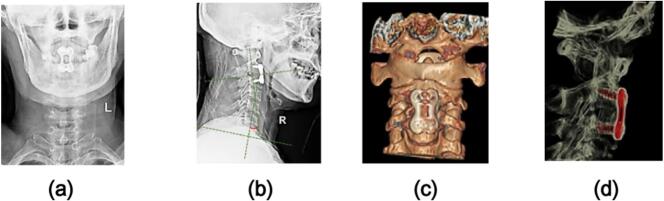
One year after operation, the patient was reexamined by cervical X-ray and CT(4a,4b,4c,4d). The Cobb angle of the injured C2-7 was improved by 16°, and the intervertebral height of the injured segment was restored by 1.8 mm compared with that at the time of injury.
